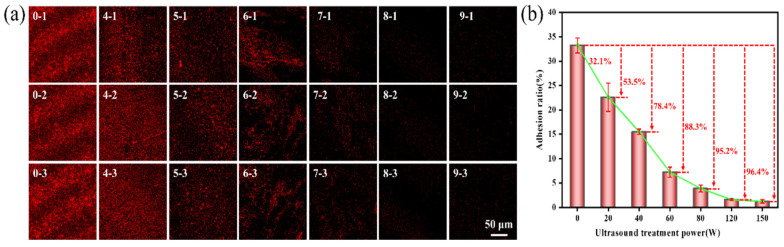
Effect of ultrasonic power on adhesion behavior of *Pseudoalteromonas*. (a) CLSM images of *Pseudoalteromonas* under different ultrasonic treatment powers: 0–1~0–3 (0 W); 4–1~4–3 (20 W); 5–1~5–3 (40 W); 6–1~6–3 (60 W); 7–1~7–3 (80 W); 8–1~8–3 (120 W); 9–1~9–3 (150 W). (b) Curve of adhesion rate of *Pseudoalteromonas* with ultrasonic treatment power.
